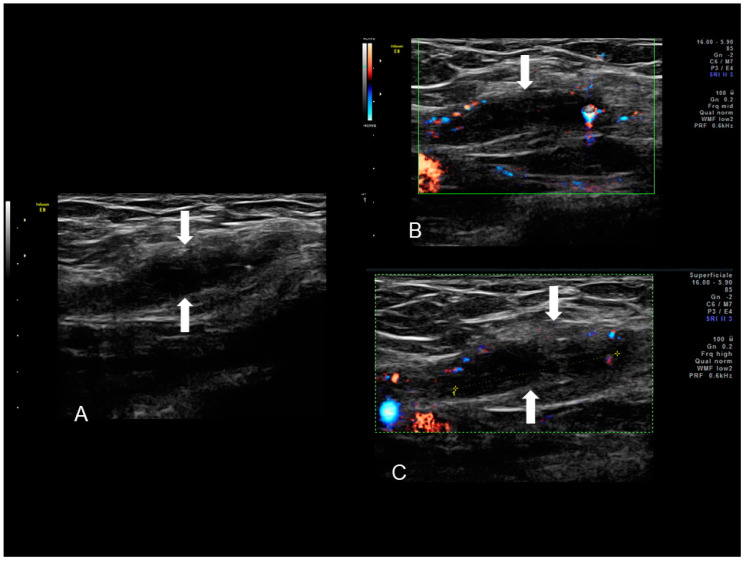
Sonographic features of right inguinal endometriosis (see white arrows) (A) presenting as a cystic mass with internal septa, hypoechoic content and few peripheral color spots located in inguinal area due to a scanty vascularization (B,C).
